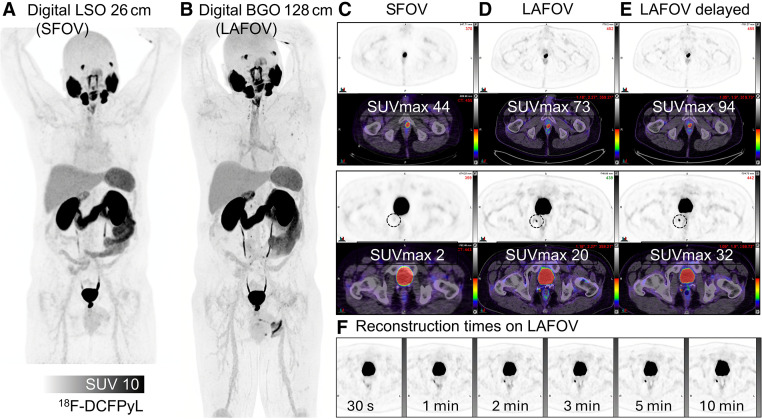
(A and B) Maximum-intensity-projection images on SFOV and LAFOV PET/CT, showing better visualization of stellate and lumbar ganglion and submucosal tracheal physiologic activity on LAFOV PET/CT. (C–E) Top: recurrence with intense uptake on all images. Bottom: <5-mm recurrence visible only on LAFOV. (F) Variable reconstruction times demonstrate clear visualization on acquisitions at 2 min and later.
